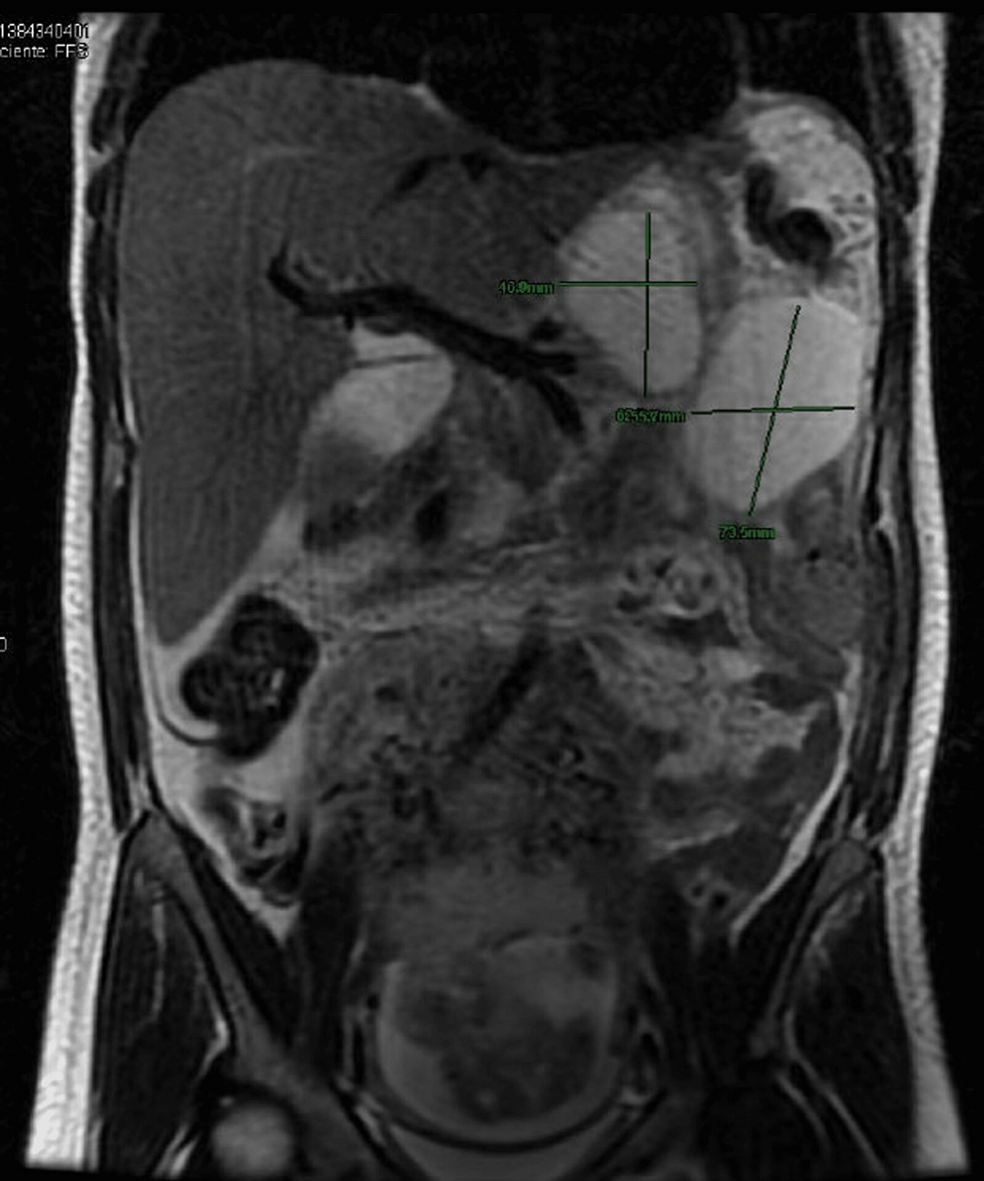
Magnetic resonance imaging of the abdomen showing peripancreatic fluid collection and splenorenal collection in the left hypochondrium.
